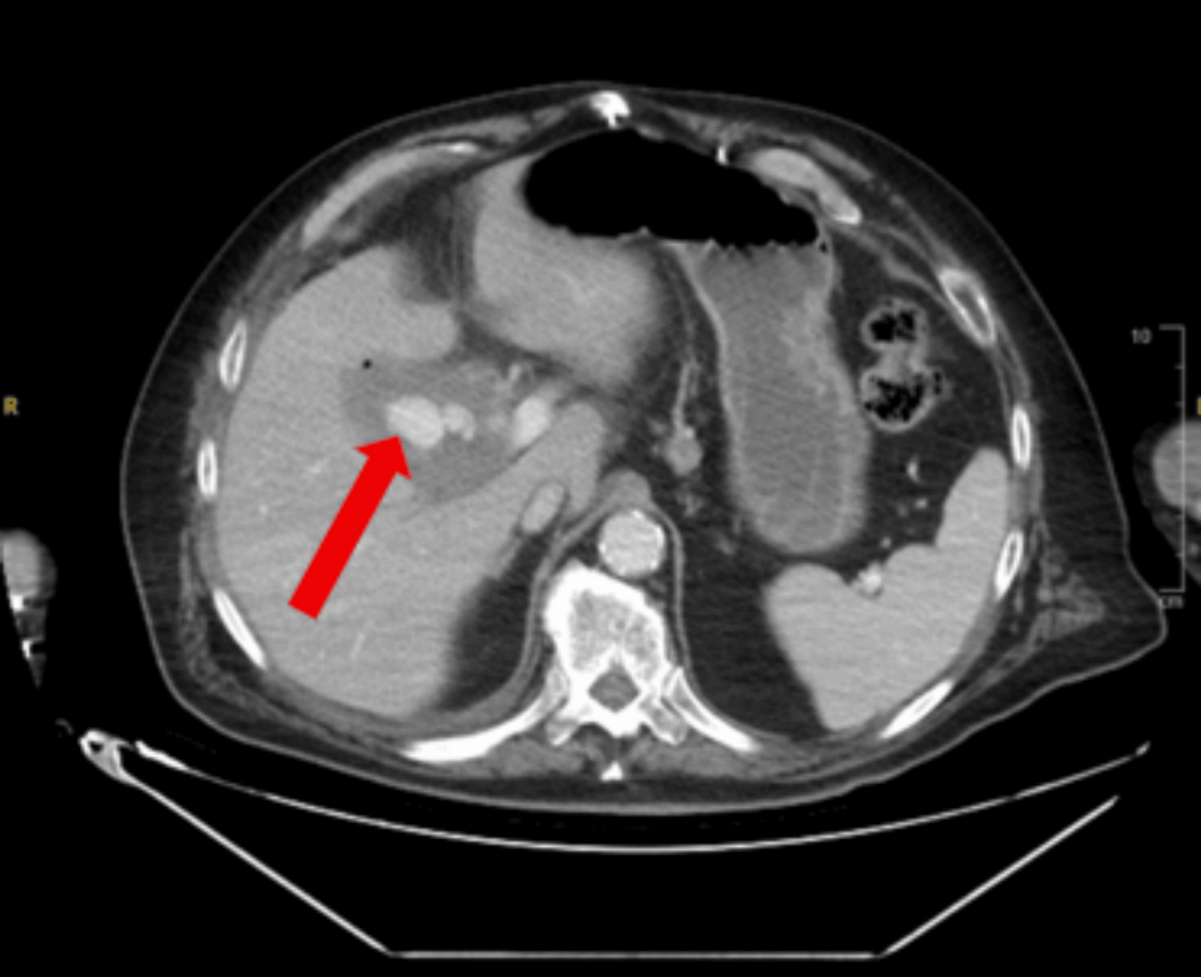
Axial CT angiogram of the abdomen and pelvis demonstrating active contrast extravasation (red arrow) from the branch of the right replaced hepatic artery into the gallbladder, showing multiple round areas of increased densities consistent with hemmorhage
